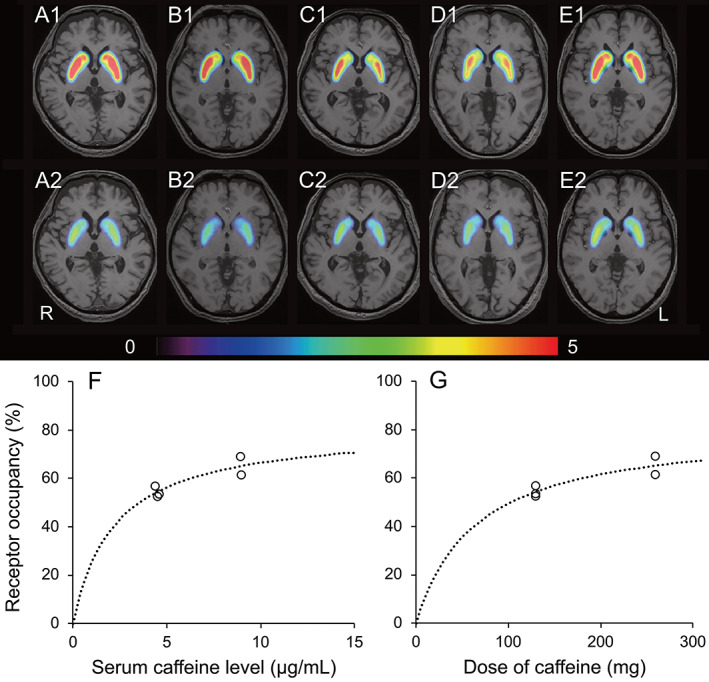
Changes in binding potential (BP_ND_) maps after caffeine intake in five patients with Parkinson's disease (A–E) and the relationships between adenosine A_2A_ receptor occupancy and serum caffeine levels (F) or caffeine dose (G). BP_ND_ maps of adenosine A_2A_ availability in patients 1 (A), 2 (B), 3 (C), 4 (D), and 5 (E) are displayed on structural magnetic resonance imaging as follows: at baseline (A1, B1, C1, D1, and E1) and after intake of coffee containing 259 mg (A2 and B2) or 129.5 mg (C2, D2, and E1) of caffeine. The rainbow‐colored scale represents the magnitude of BP_ND_ values. Patients 1–5 correspond to the numbers of the five patients in Table 1. The dashed curve was modeled using the following equation: occupancy (%) = α × [D/(D + ED_50_)], where α refers to the maximal receptor occupancy, D refers to serum caffeine levels (F) or caffeine dose (G), and ED_50_ refers to the level resulting in 50% of maximal receptor occupancy. L, left; R, right. [Color figure can be viewed at wileyonlinelibrary.com]
